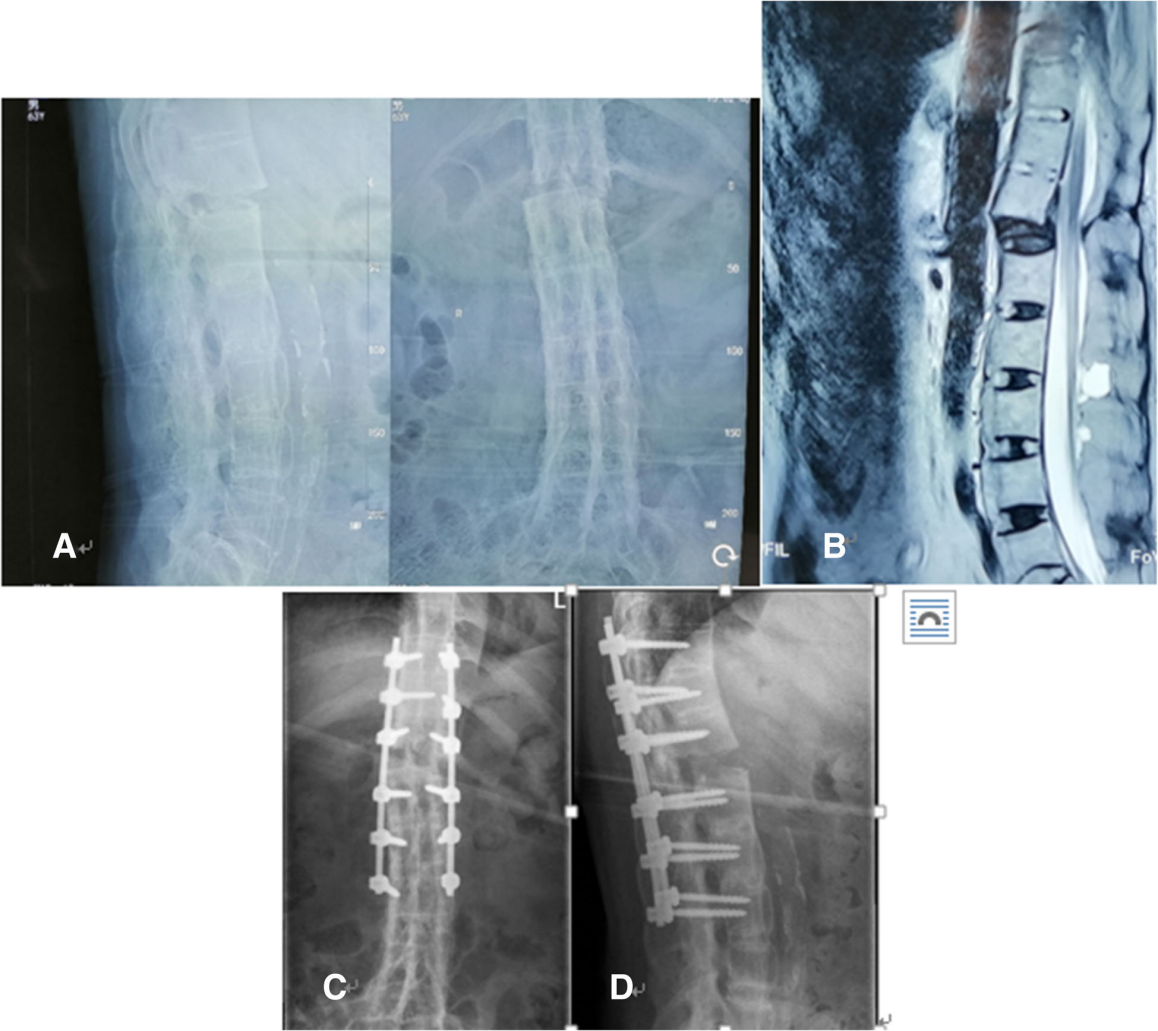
The patient had a 40-year history of AS and was admitted to the hospital due to a fall. a Preoperative X-ray shows an obvious intervertebral bone bridge made a “bamboo-like spine”, and the fracture end is obviously displaced. b Preoperative MRI also shows the fracture end is obviously displaced. c-d Postoperative X-ray shows the second pedicle screw on the right side did not meet the ideal position, resulting in the urinary dysfunction after operation, which recovered 3 months after discharged. And his neurological function was improved from degree D to degree E 1 year after surgery
